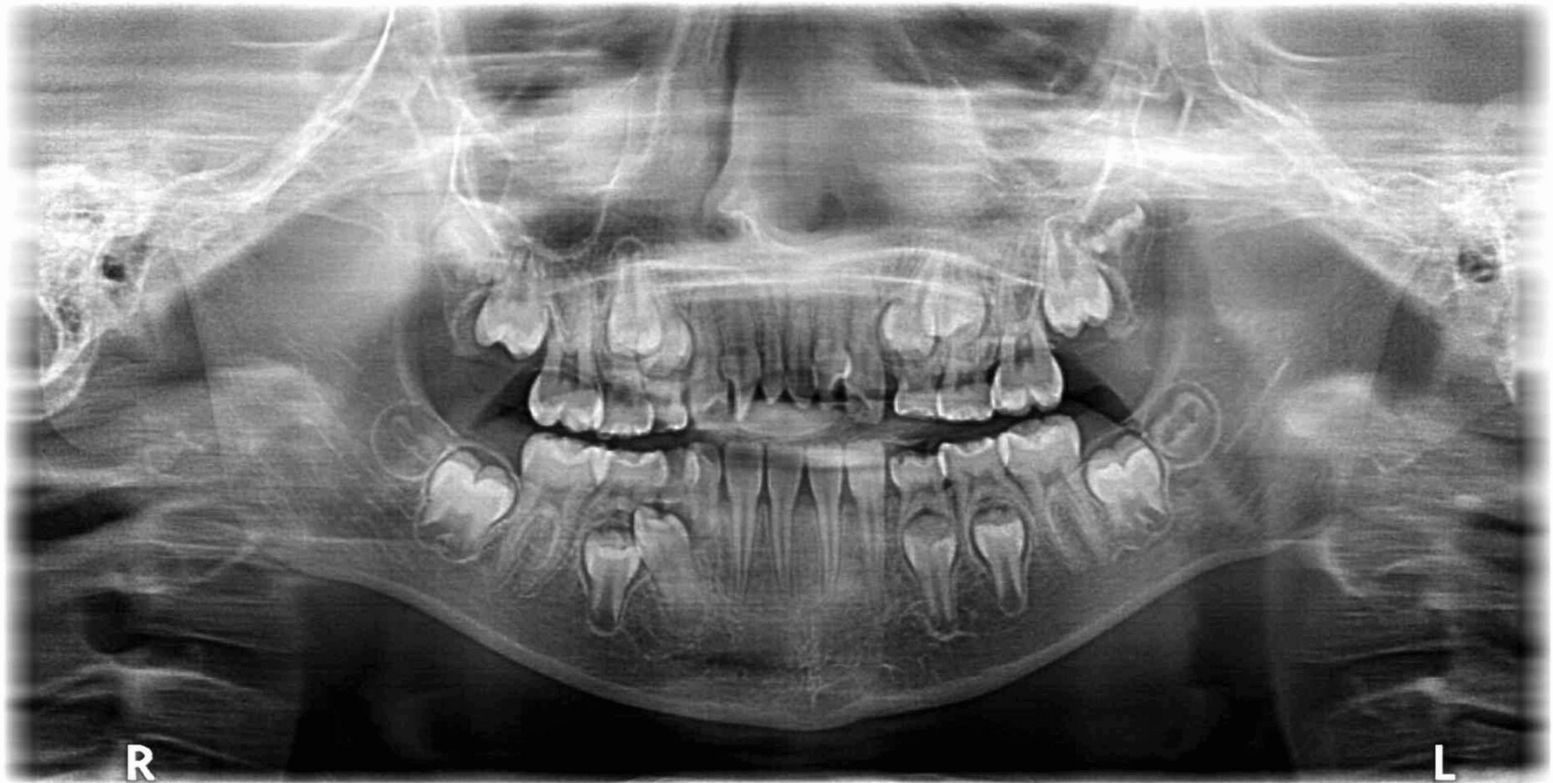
Panoramic radiograph of the patient
